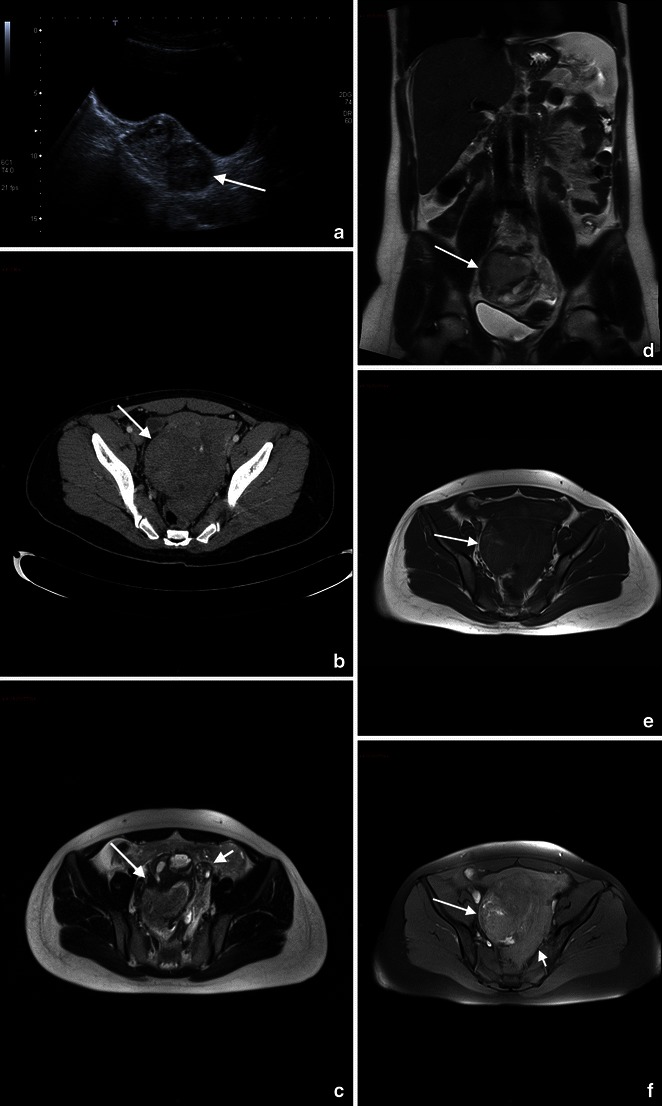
A 27-year-old woman presenting at the emergency department with acute pelvic pain. Abdominal US (a) shows a rounded lesion adjacent to the uterus, clearly separate from the left ovary. Axial CT scan shows a voluminous mass in the pelvic cavity (b); for the characterisation an MRI was required. Axial (c) and coronal (d) T2-weighted and T1-weighted images (e) of the pelvis show a right heterogeneous adnexal mass (arrow) with fallopian tube haematoma. Note the normal ovary (short arrow) in (c). Pre-contrast T1-weighted fat-saturated image (f) shows bloody ascites (short arrows). These findings are due to ectopic pregnancy with tubal rupture and haemoperitoneum
